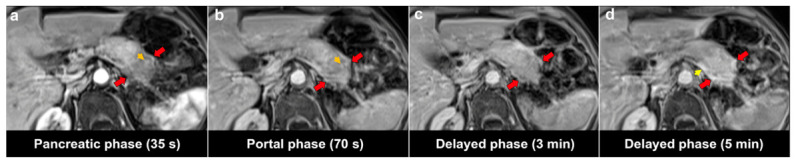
Focal AIP with segmental involvement of the pancreatic body and tail, showing progressive delayed enhancement on dynamic contrast-enhanced MRI. (a,b) Axial images in the pancreatic and portal phases show reduced uptake in the affected pancreas (red arrows), with dotted or speckled areas of enhancement (orange arrowheads) representing spared normal parenchyma. (c,d) Axial images in the delayed phases, acquired 3 and 5 min after contrast administration, demonstrate progressive homogeneous enhancement of the involved pancreas (red arrows). Note also the progressive enhancement of the peripancreatic “halo”, which becomes more conspicuous on the 5 min delayed phase (yellow arrowhead).
